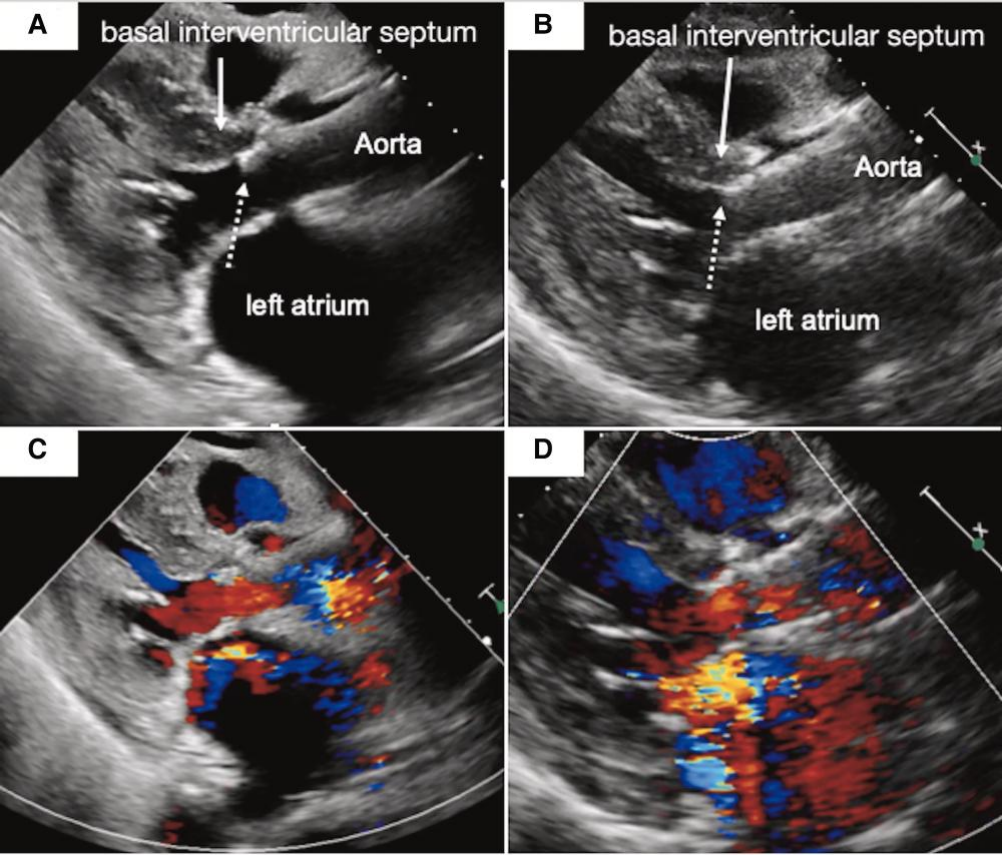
Echocardiographic views. (*A*) Post-transcatheter heart valve 2D-mode image. (*B*) Corresponding pre-transcatheter heart valve colour Doppler image. (*C*) Post-transcatheter heart valve transthoracic echocardiographic 2D-mode image obtained during heart failure exacerbation. Marked reverse remodelling of the left ventricular myocardium was observed. (*D*) Corresponding post-transcatheter heart valve colour Doppler image. The dotted arrow indicates the leftward migration of the transcatheter aortic valve implantation stent frame into the left ventricle.
